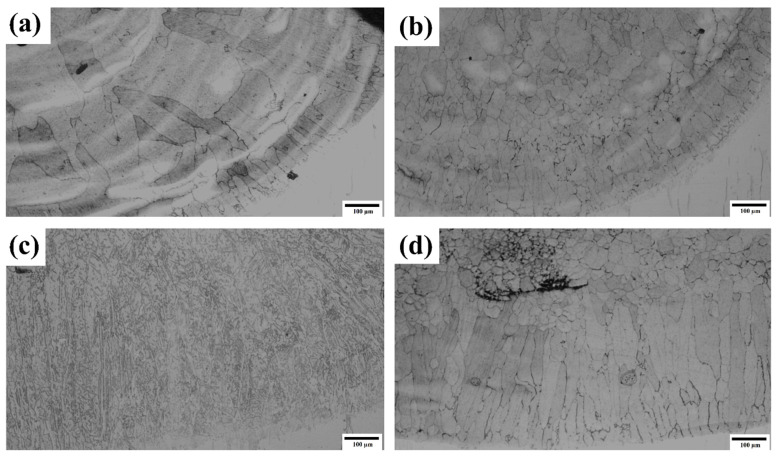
Microstructure of HEA R-UVALC coating near the substrate (a) without ultrasonic, (b) with ultrasonic of 5 µm, (c) with ultrasonic of 10 µm, and (d) with ultrasonic of 15 µm.
